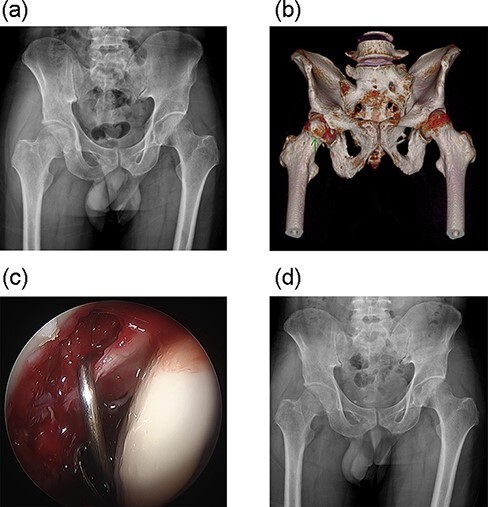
A 40-year-old man (Case 17) was transferred from other hospital due to persisted hip pain even after 3 months’ conservative treatment. (a) The radiology of the right hip showed incongruent joint with bony fragments. (b) 3D-CT showed that the fragments of Pipkin Type I femoral head fracture were small and comminuted and were excised. (c) The intercalated bone fragment that made joint incongruent was removed by arthroscopy. (d) The radiology showed symmetric hip joint space after the removal of bony fragment.
